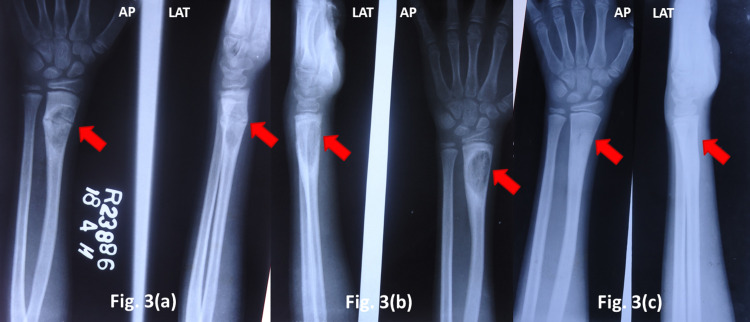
TB of distal end radius (a) Plain radiograph at presentation (b) After eight months on ATT (c) At 18 months follow-up TB - tuberculosis, ATT - anti-tubercular drug therapy
